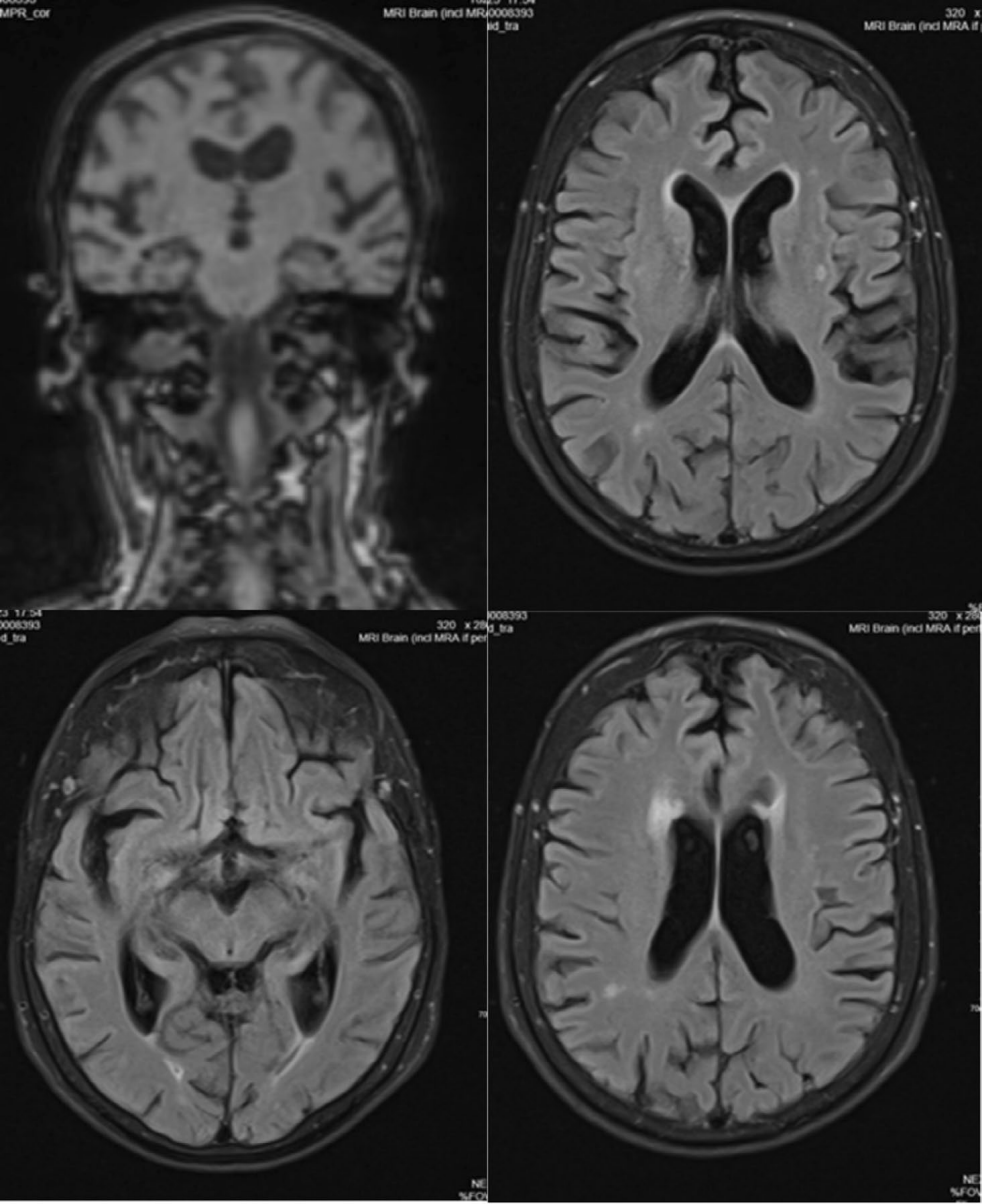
MRI brain‐fluid‐attenuated inversion recovery (FLAIR) sequences (showing periventricular WMHs).
